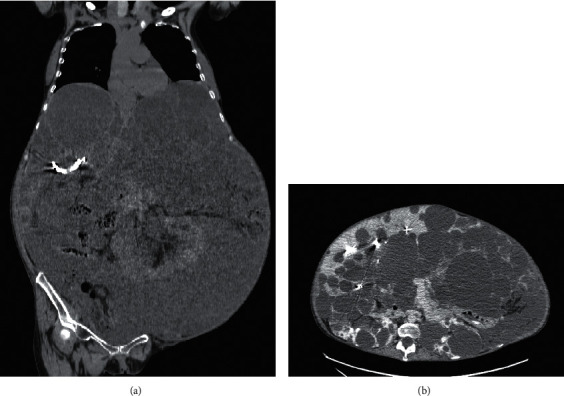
Preoperative images of computed tomography showing a massively enlarged polycystic liver that occupied the abdominal space and compressed the diaphragm and abdominal wall. (a) Coronal section. (b) Axial section.
